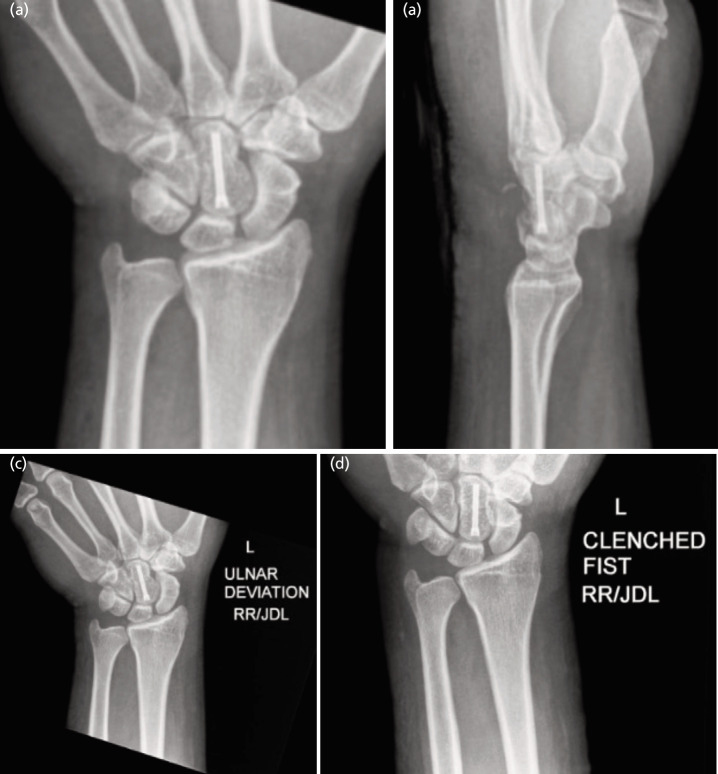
Post-operative radiographs of the hand. (a) PA, (b) lateral, (c) ulnar deviation, (d) clenched fist) confirming the successful reduction and proper alignment of the capitate after open reduction and internal fixation (ORIF) with screw fixation. The PA of the wrist with ulnar deviation and clenched fist demonstrates proper alignment of the scaphoid and lunate, with no evidence of diastasis or abnormal gapping, indicating preserved scapholunate ligament integrity with the scapholunate interval maintained within the normal range of 0.25mm (N .3mm).
